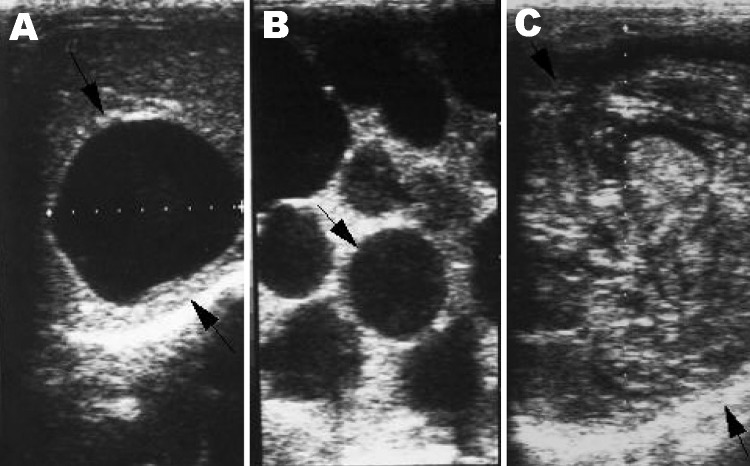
Lesions of cystic echinococcosis (CE) by abdominal ultrasound examination. A) CE lesion with distinct rim. B) Typical CE lesion with daughter cysts. C) Calcified CE lesion after chemotherapy.
